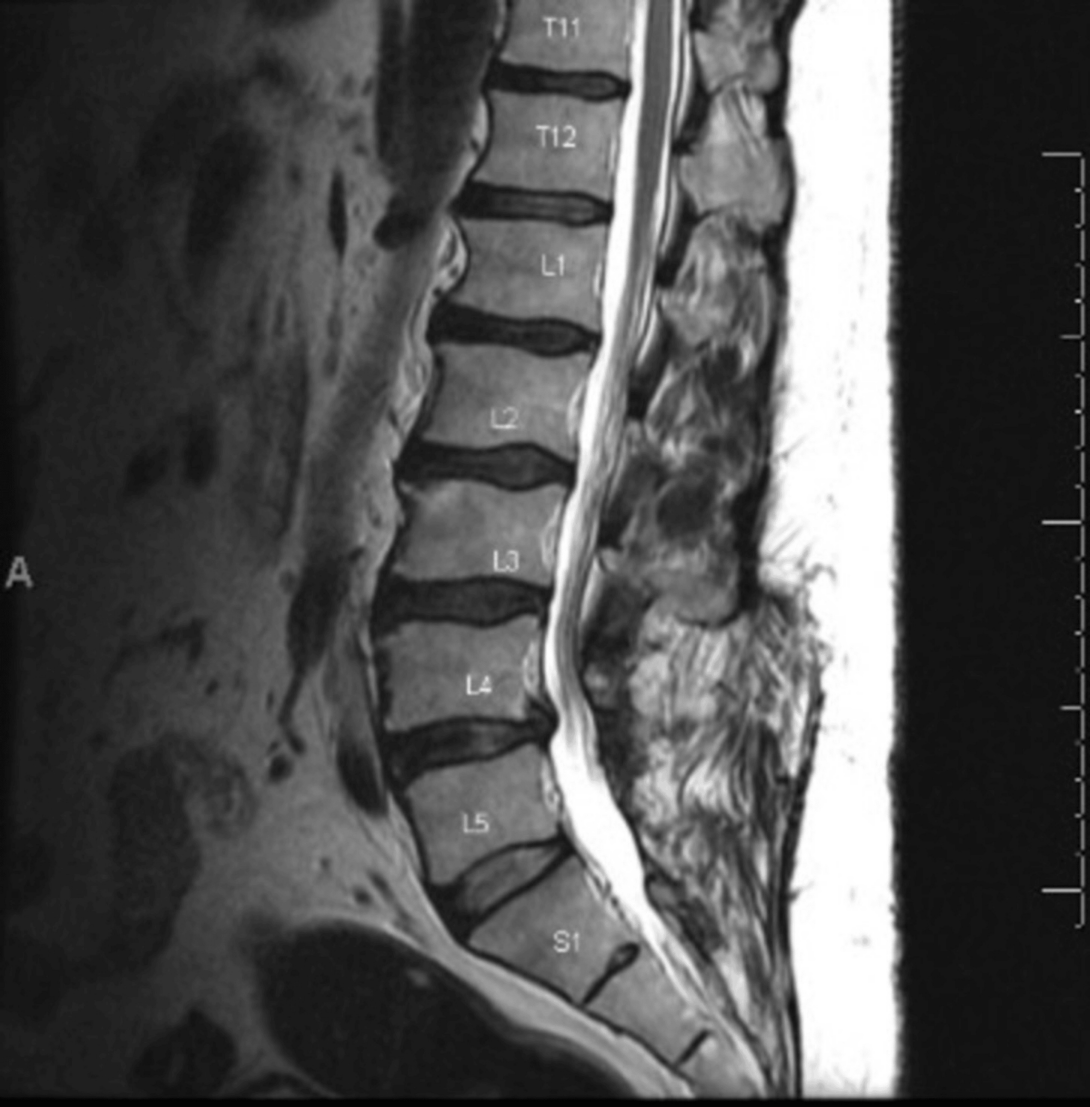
Sagittal MRI view of the lumbar spine for Case 2 following surgical decompression.
